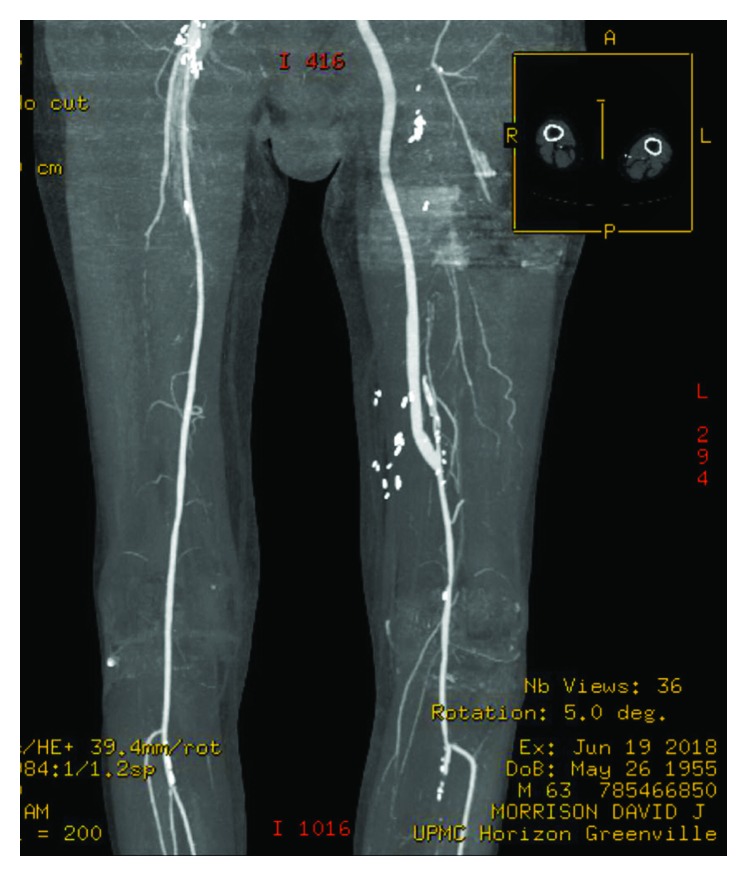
Postoperative CT angiogram of obturator bypass demonstrating distal anastomosis and runoff.
